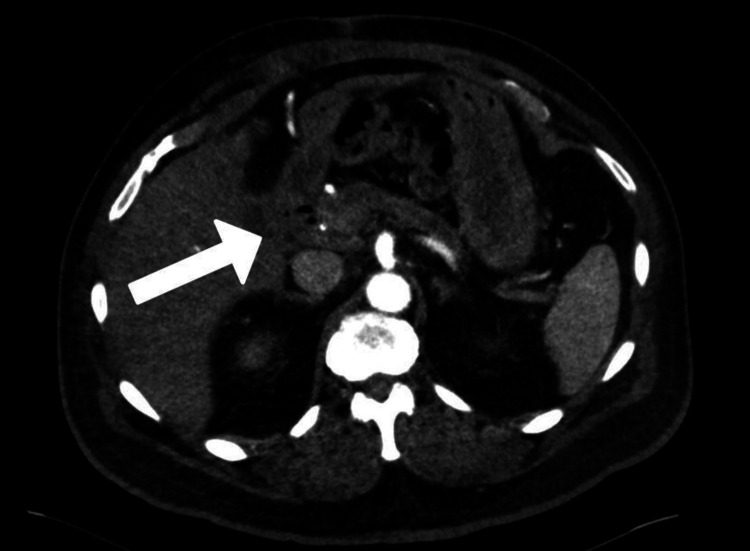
CT pancreas depicting pancreatic head mass (arrow) with marked dilation of the main pancreatic duct and pancreatic tail atrophy. CT: Computed tomography
